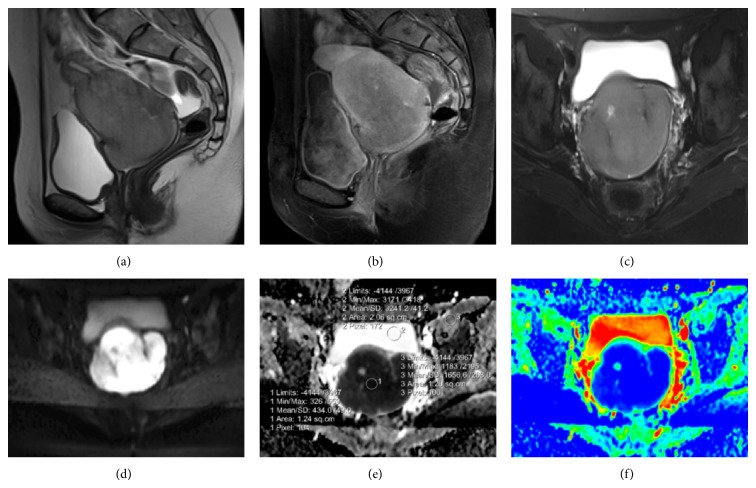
(a) Sagittal T2-weighted imaging shows a slightly hyperintense mass confined to the cervix. Free intraperitoneal fluid is evident. (b) Sagittal postcontrast T1-weighted imaging displays heterogeneous enhancement of the mass. (c) Cystic area is seen on axial T2 fat suppressed image. (d) Axial TRACE images reveal prominent diffusion restriction in the mass lesion. (e, f) Corresponding ADC and coloured ADC maps confirm high cellularity.
